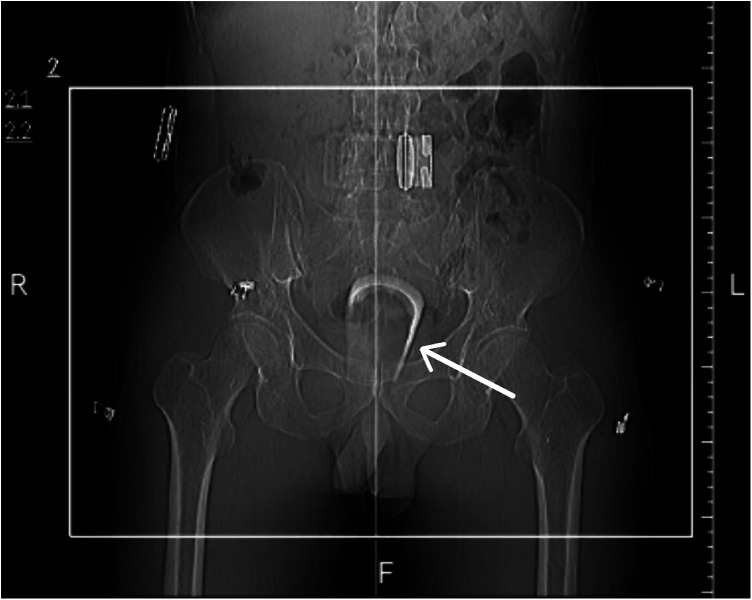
Plain abdominal X-ray film revealed an irregular dense shadow approximately 7 cm in length within the pelvis (white arrow).
